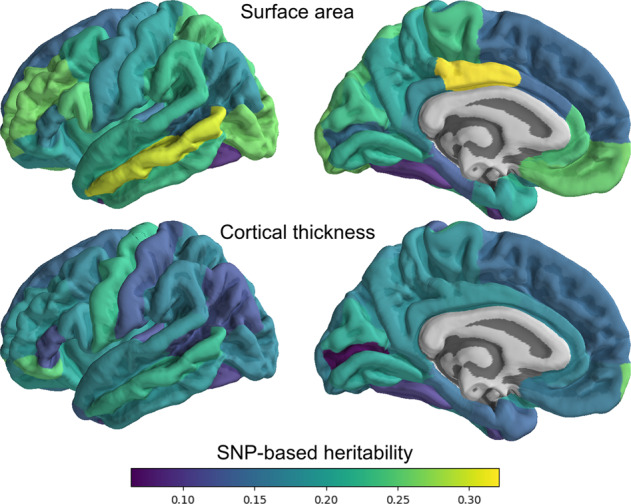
Regional SNP-based heritability across the cortex, with the lateral (left) and medial (right) views for surface area at the top and cortical thickness at the bottom row. The estimates are calculated through LD score regression [132], applied to MRI data from 33,735 White European individuals as described previously [115], and parcellated according to the widely-used Desikan Killiany atlas [133]. As indicated through the colour-coding, heritability ranges from below 0.10 to above 0.30, with the regional estimates for surface area showing a wider spread than cortical thickness.
